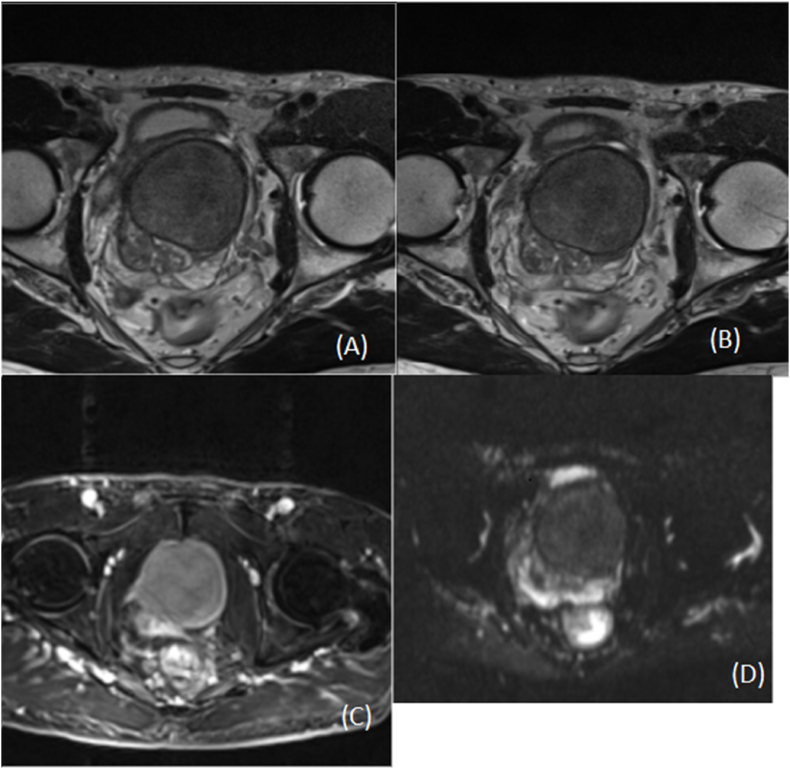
Magnetic resonance imaging showing an enlarged prostate (154.3 cc; 162 g) with superior bulging over the bladder floor. The peripheral zone presents normal diffusion and ADC values, with mildly heterogeneous T2 signal. The transition zone shows encapsulated nodules, with the largest located on the left (6.1 × 5.4 × 5.9 cm). Panel (A): anatomical image (T2-weighted sequence); panels (B) and (C): diffusion sequences; panel (D): perfusion.
